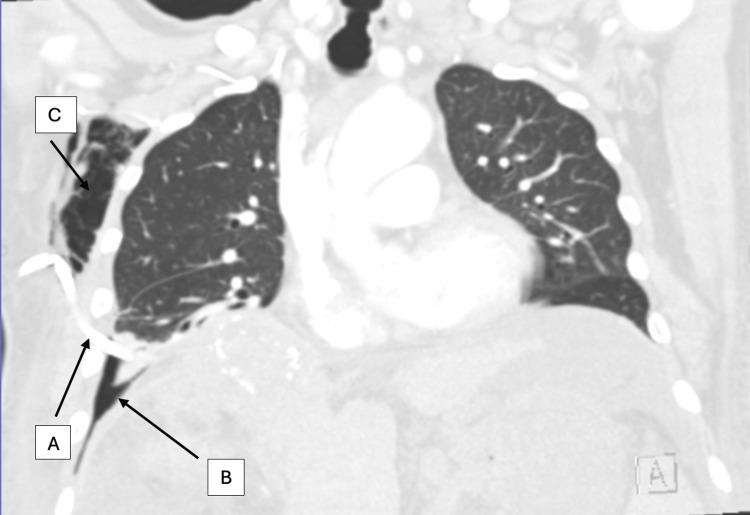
CT scan of the chest (lung view): (A) Chest tube in place and (B) hydropneumothorax with small left pleural effusion and near complete atelectasis of the right lower lobe. (C) Moderate amount of right chest wall subcutaneous emphysema.
